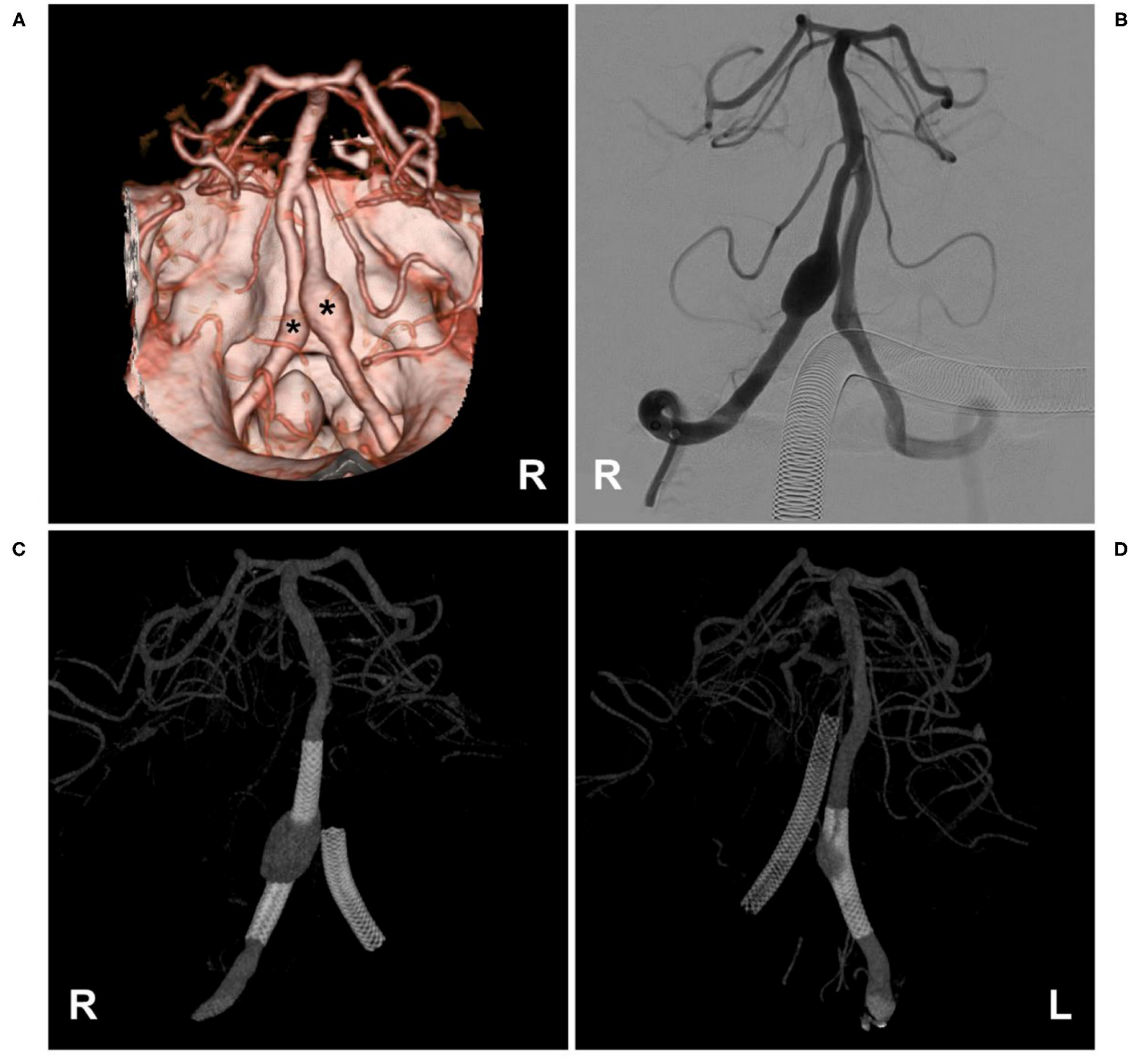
Flow diversion deployment to treat bilateral VA aneurysms. (A,B) CTA (A) and VA angiogram (B) showing bilateral VA dissecting aneurysms (asterisks in (A)); in (B), the ASA was not observed. (C,D) Angiogram of the bilateral VA showing the flow diversions deployed to treat the aneurysms. ASA, anterior spinal artery; CTA, computed tomography angiography; L, left; R, right; VA, vertebral artery.
